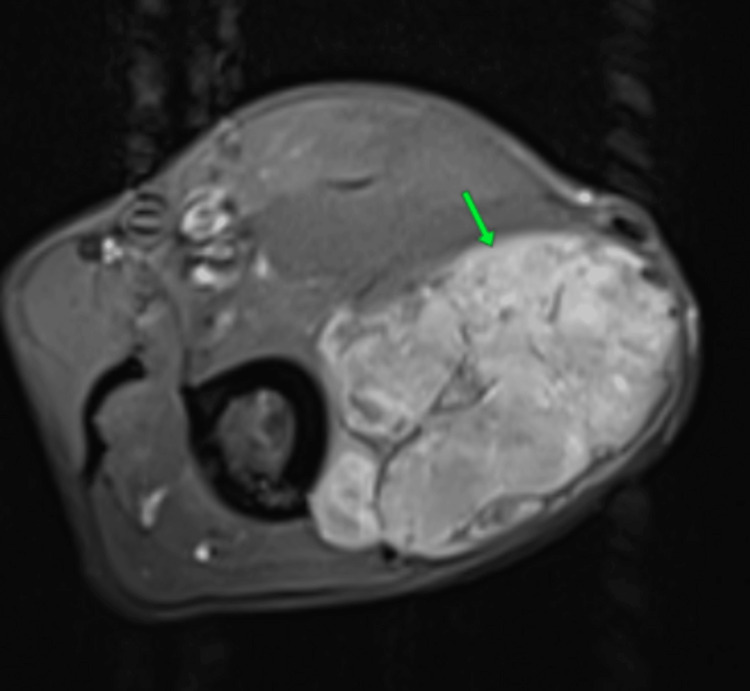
Axial MRI of the arm showing the presence of a mass involving the lateral head of the biceps muscle, hyperintense on T1 with homogeneous enhancement after gadolinium injection (green arrow).
